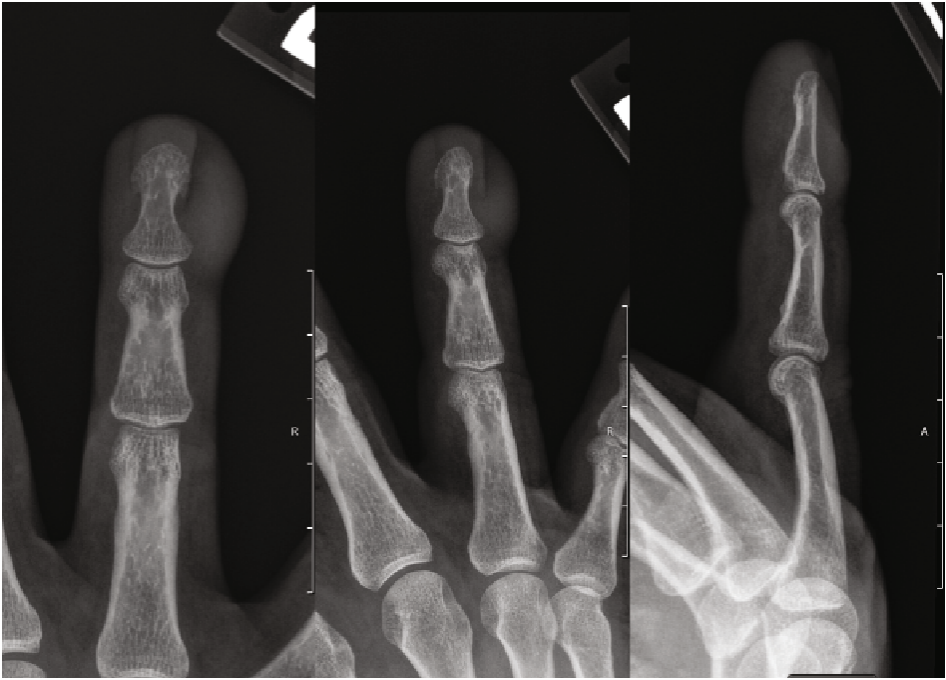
Three-view x-ray. PA, lateral, and oblique views from left to right.
